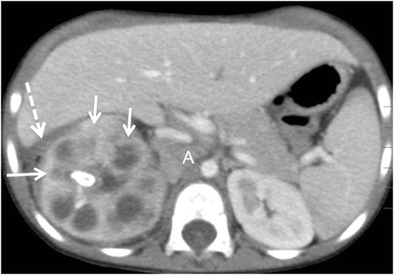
Diffuse XPN. Contrast-enhanced nephrogenic phase transverse CT shows enlarged right kidney with hypodense areas with peripheral enhancement (*white arrows*), a stone in the pelvis and extrarenal extension (*discontinuous arrows*). Adenopathy (*A*) displacing cava vein is present
